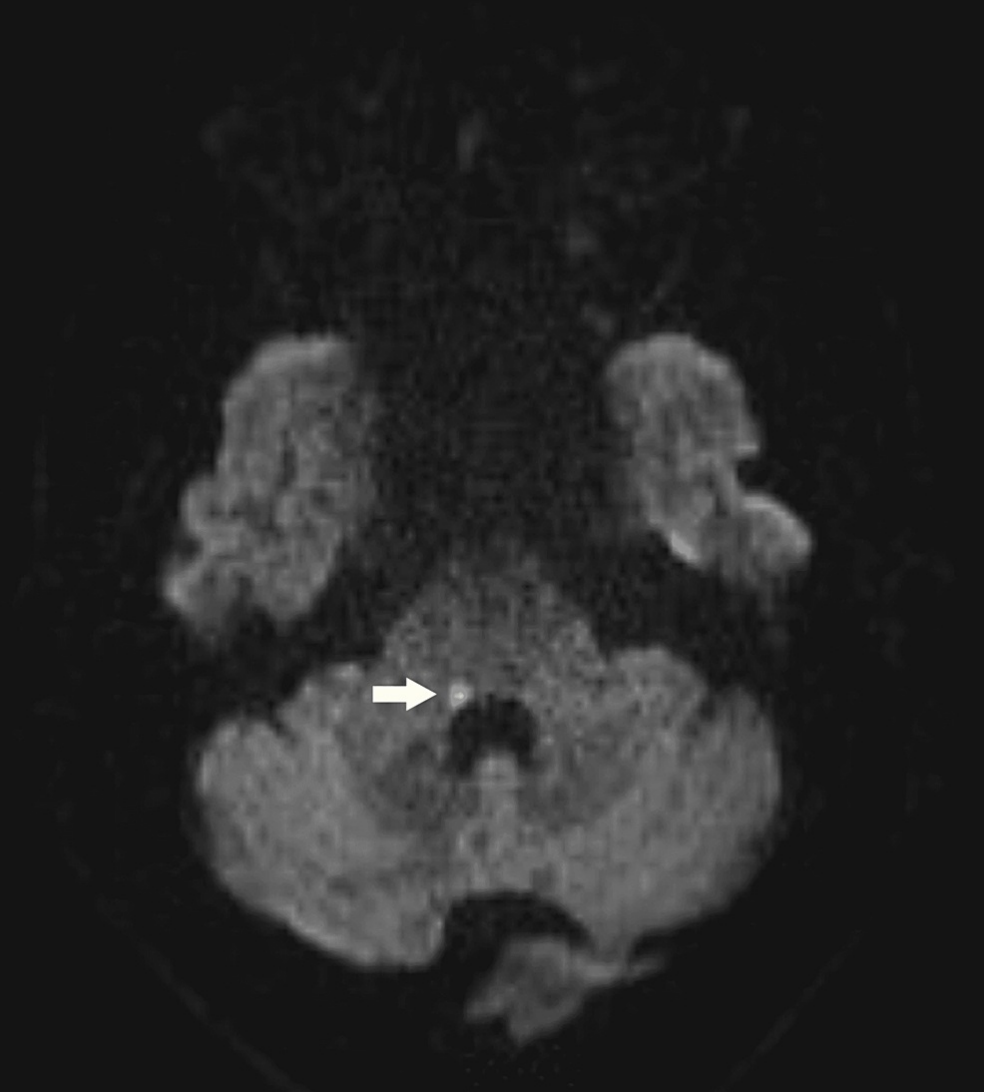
MRI Brain DWI sequence with arrow showing an acute infarct in the right facial colliculus
